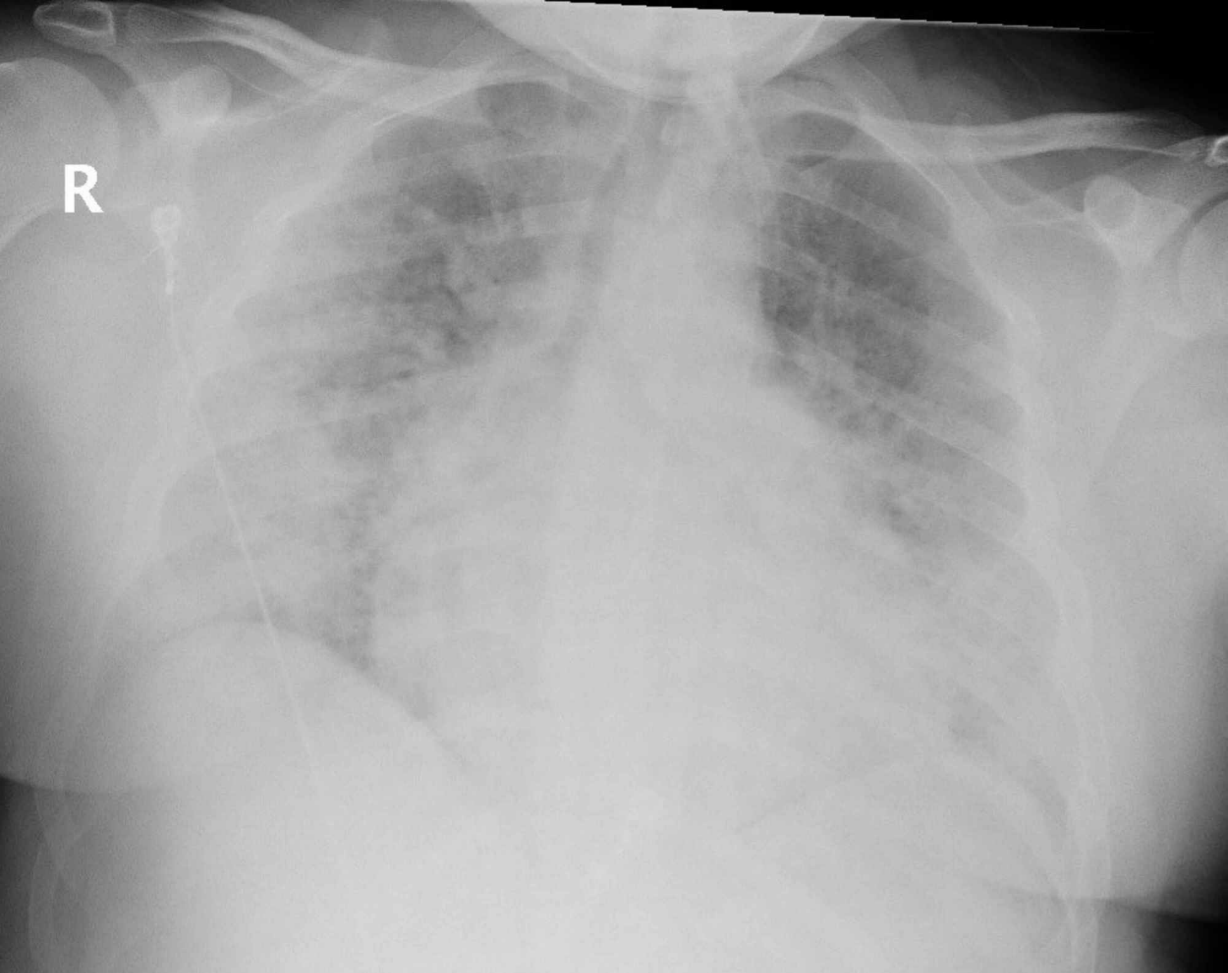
Case 2 chest X-ray on March 26, 2020
